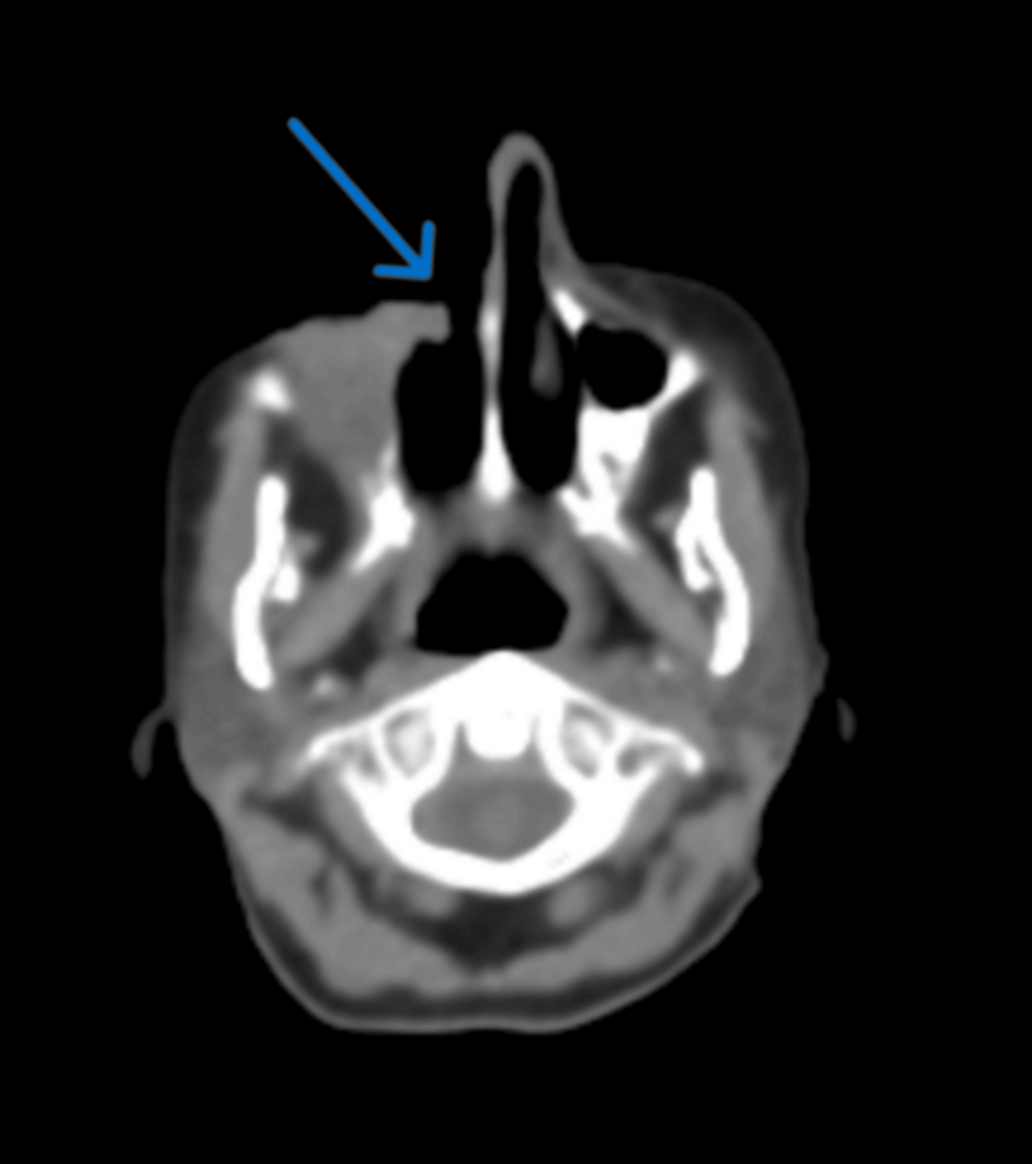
Axial post-treatment CT scan of the head and neck demonstrating tumor regression. Follow-up contrast-enhanced CT of the head and neck showed a significant decrease in the size of the previously noted right facial mass, approximately 45%-50% reduction in maximal tumor diameter. The tumor's extension into the orbit and oral cavity has regressed, indicating a favorable partial response to systemic therapy.
